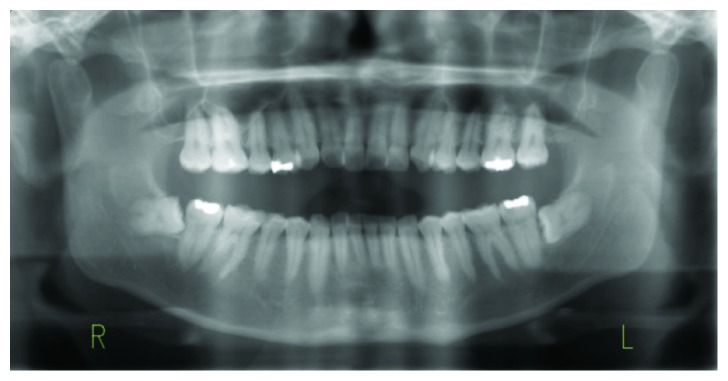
A panoramic radiograph obtained before the extraction. The radiograph shows that the right lower third molar was horizontal (Pell and Gregory Class I, position A). There are no prior bone lesions associated with the molar, such as cyst and tumor.
